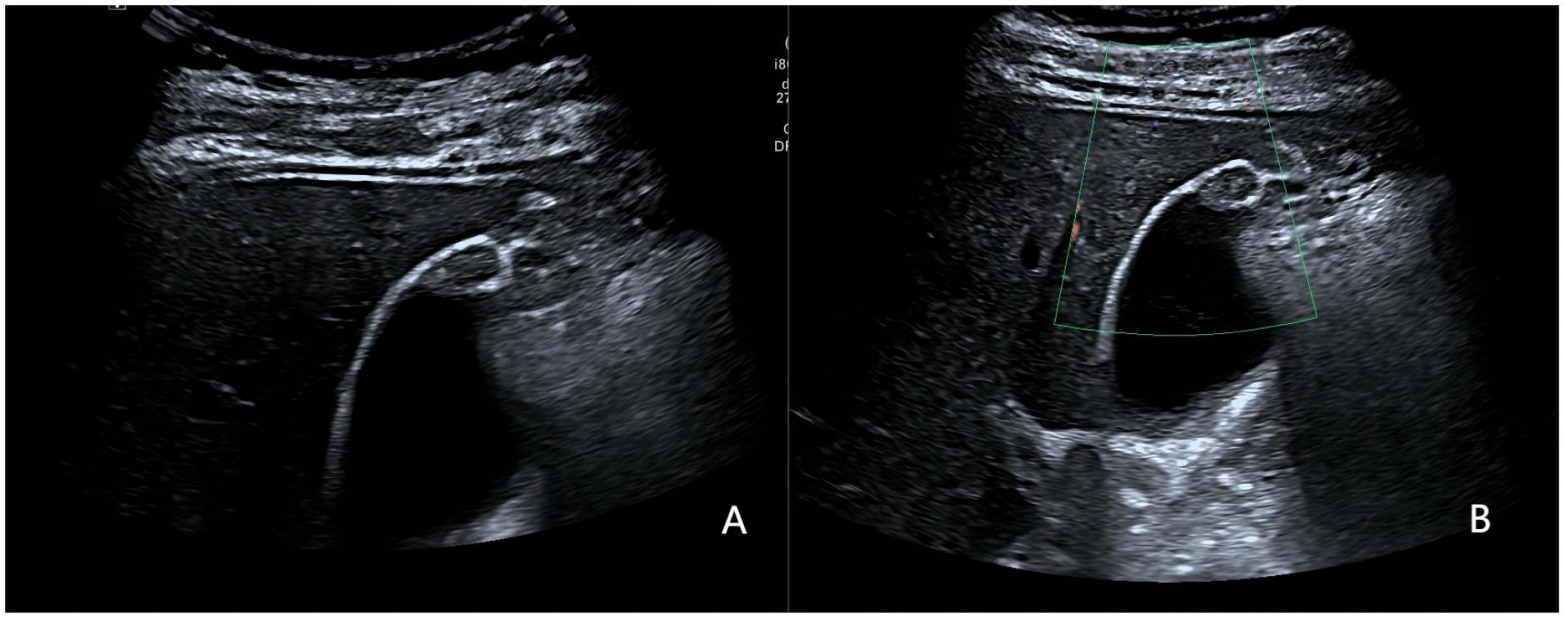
A 36-year-old woman with pathologically proven xanthogranulomatous cholecystitis. (A) Focak thickening of the base of the gallbladder with layering; (B) CDFI=0.Based on a consensus review of the sonographic findings, the lesion was categorized as GB-RADS 2, GB-RADS+CDFI 2.
